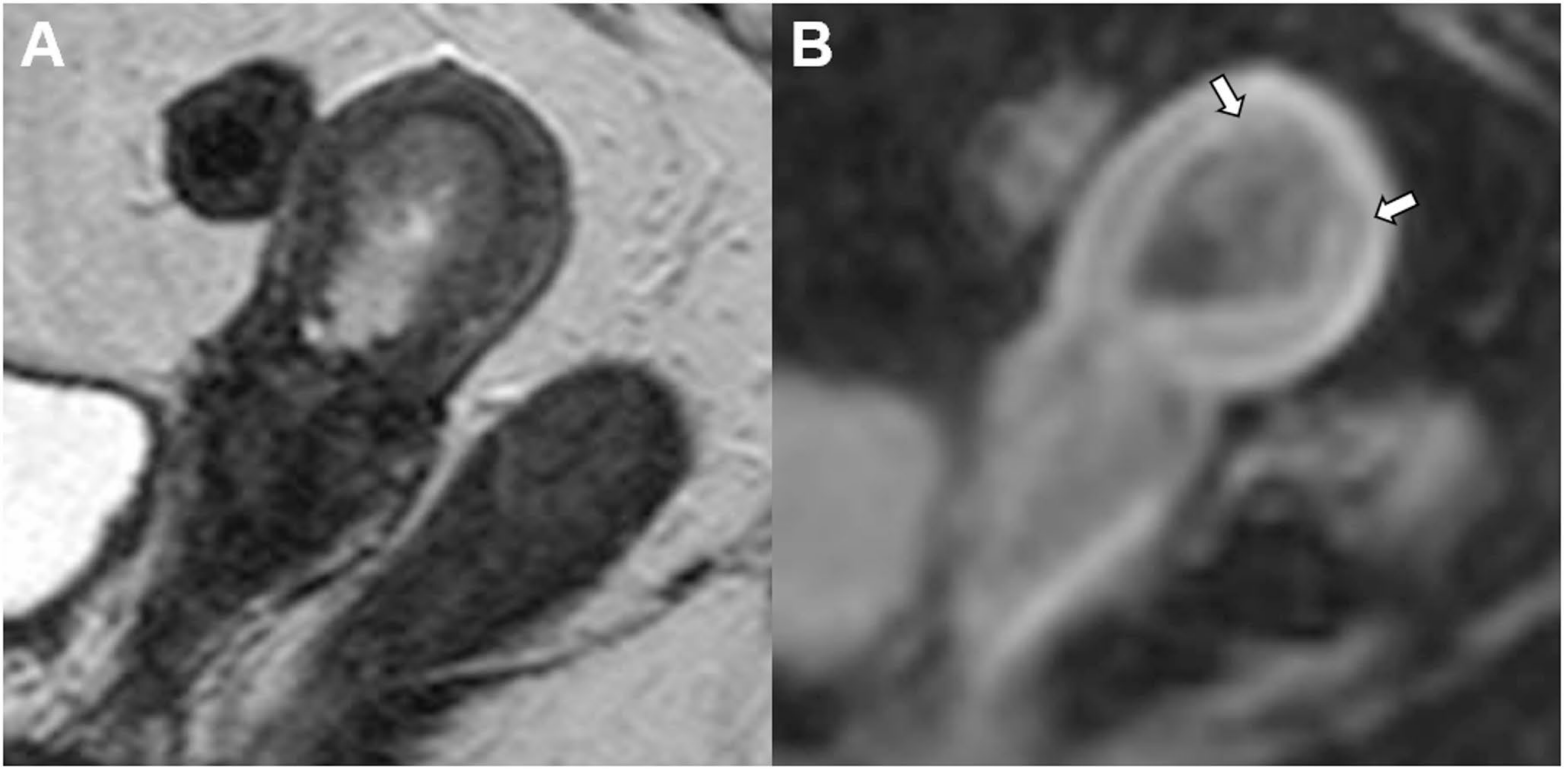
Stage IA2 grade 2 endometrioid carcinoma, no LVSI, and myometrial invasion < 50% in a 58-year-old woman. A Sagittal T2-weighted fast spin-echo (FSE) image (TR/TE: 4500/100 ms; slice thickness: 3 mm) and B contrast-enhanced 3D T1-weighted fat-suppressed LAVA sequence (TR/TE: 4.2/2.1 ms; slice thickness: 2 mm; post-gadolinium, delayed phase at 4 min) show an endometrial mass with superficial myometrial invasion (arrows)
